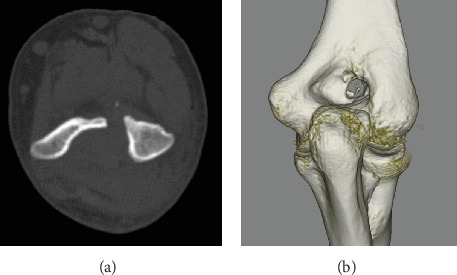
Postoperative computed tomography (CT). (a) Axial and (b) three-dimensional CT images show appropriate resection of the nidus.
